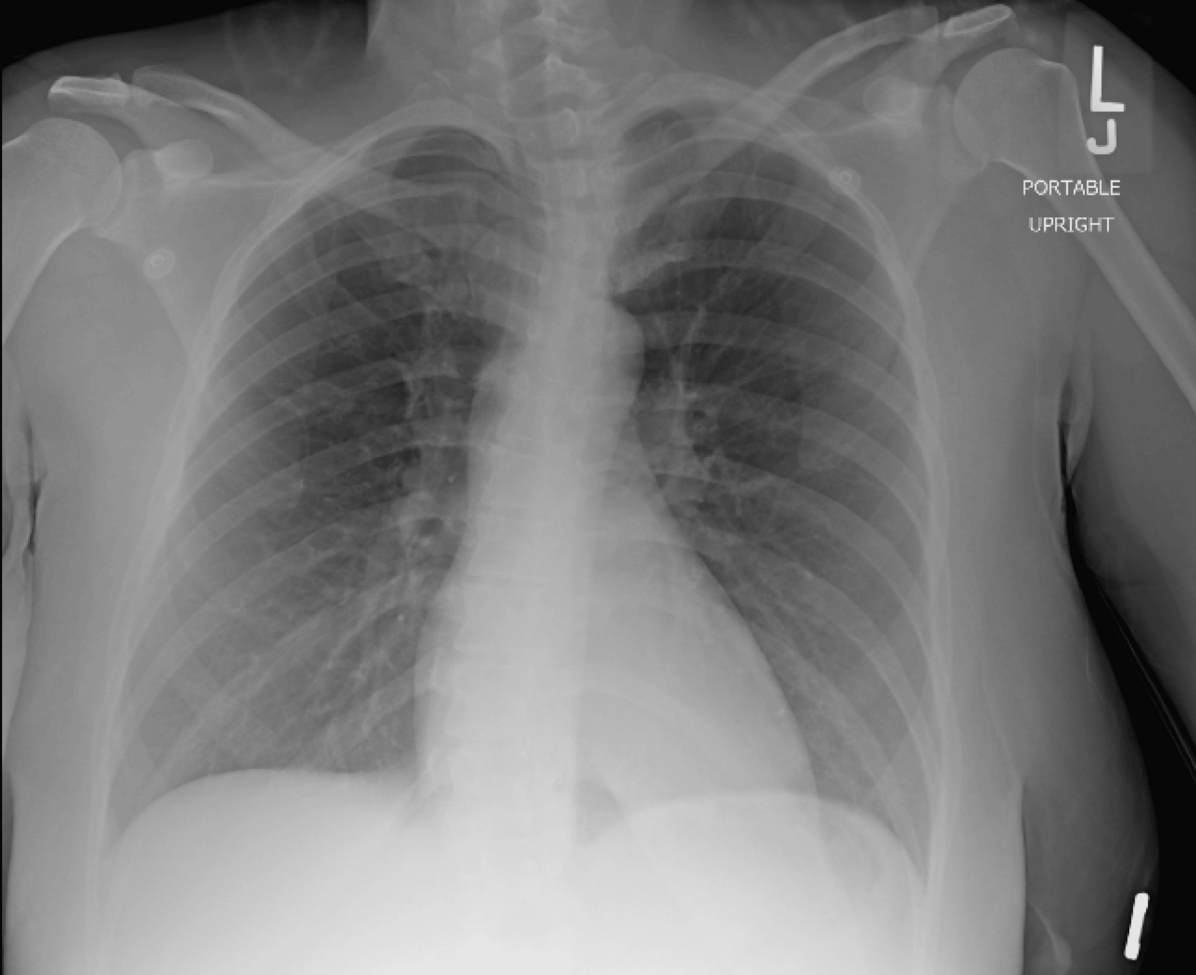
Chest X-ray obtained on admission was negative for any acute cardiopulmonary process
